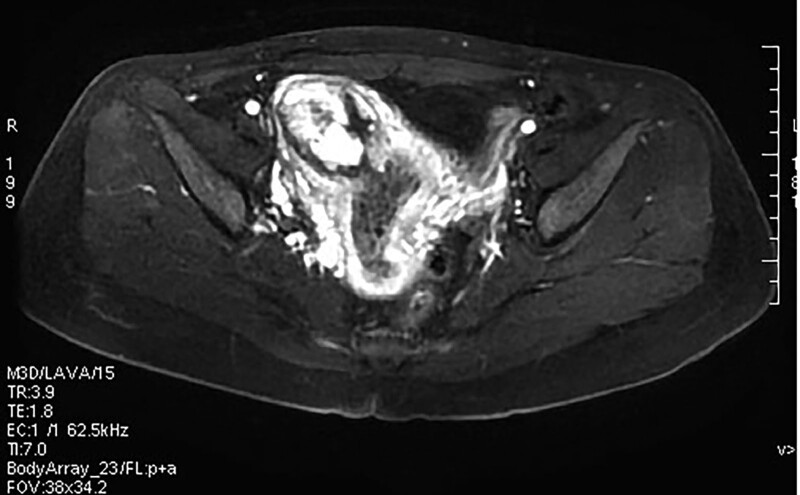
Magnetic resonance image of uterine horn lesions.
